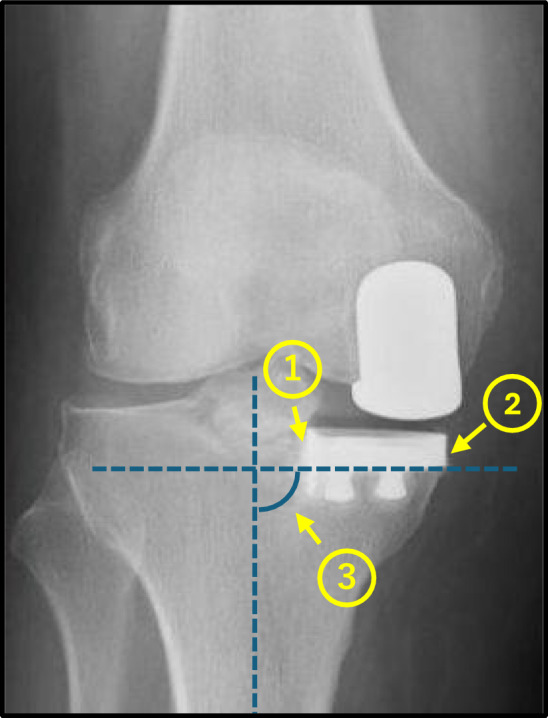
Clinical evaluation parameters on postoperative UKA X-rays: ① observation of loosening in the gap between the interbone eminence and the tibial baseplate, ② assessment of the positional relationship between the medial edge of the tibial baseplate and the medial edge of the tibia, ③ measurement of the tibial baseplate varus angle relative to the tibial axis
